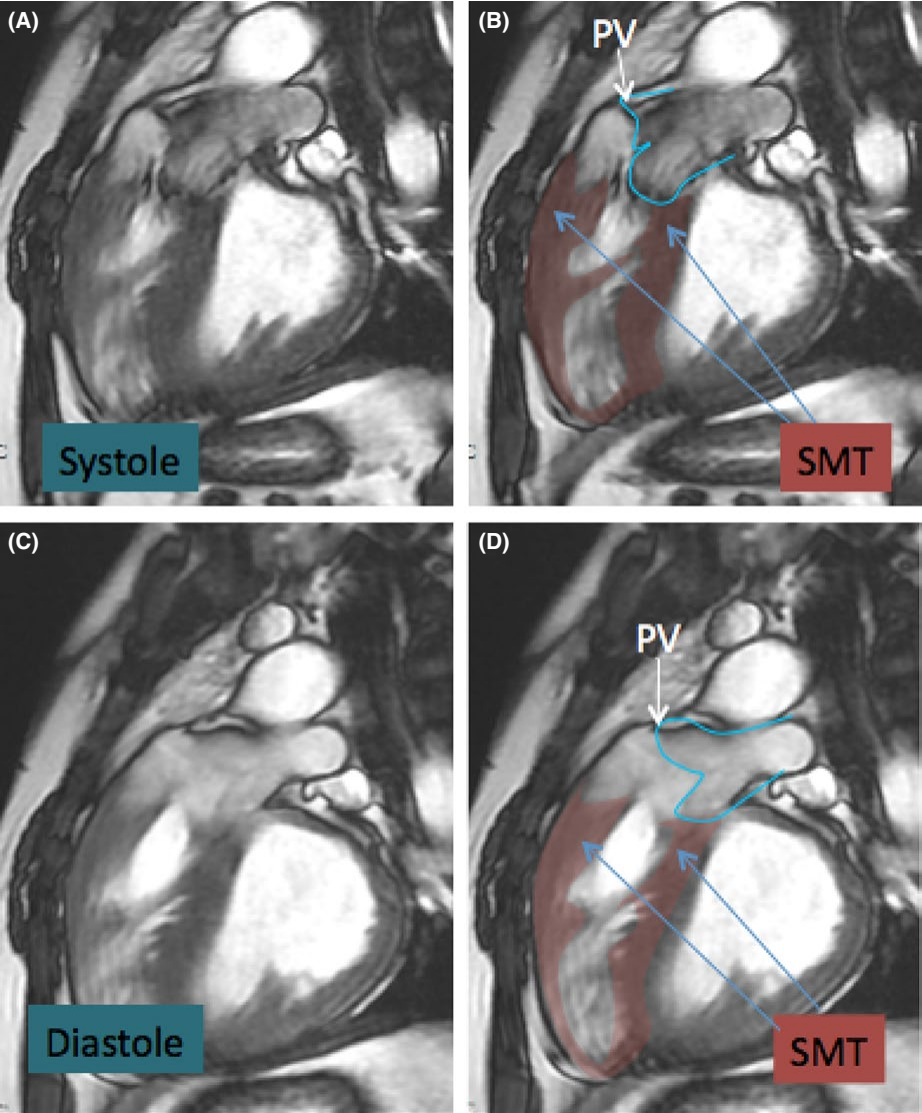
Long‐axis view of cardiac magnetic resonance imaging (MRI). Panel A and B showing right ventricular outflow tract (RVOT) subpulmonic stenosis during systole with pulmonary valve (PV) and septomarginal trabeculation (SMT). Panel C and D showing RVOT subpulmonic stenosis during diastole
